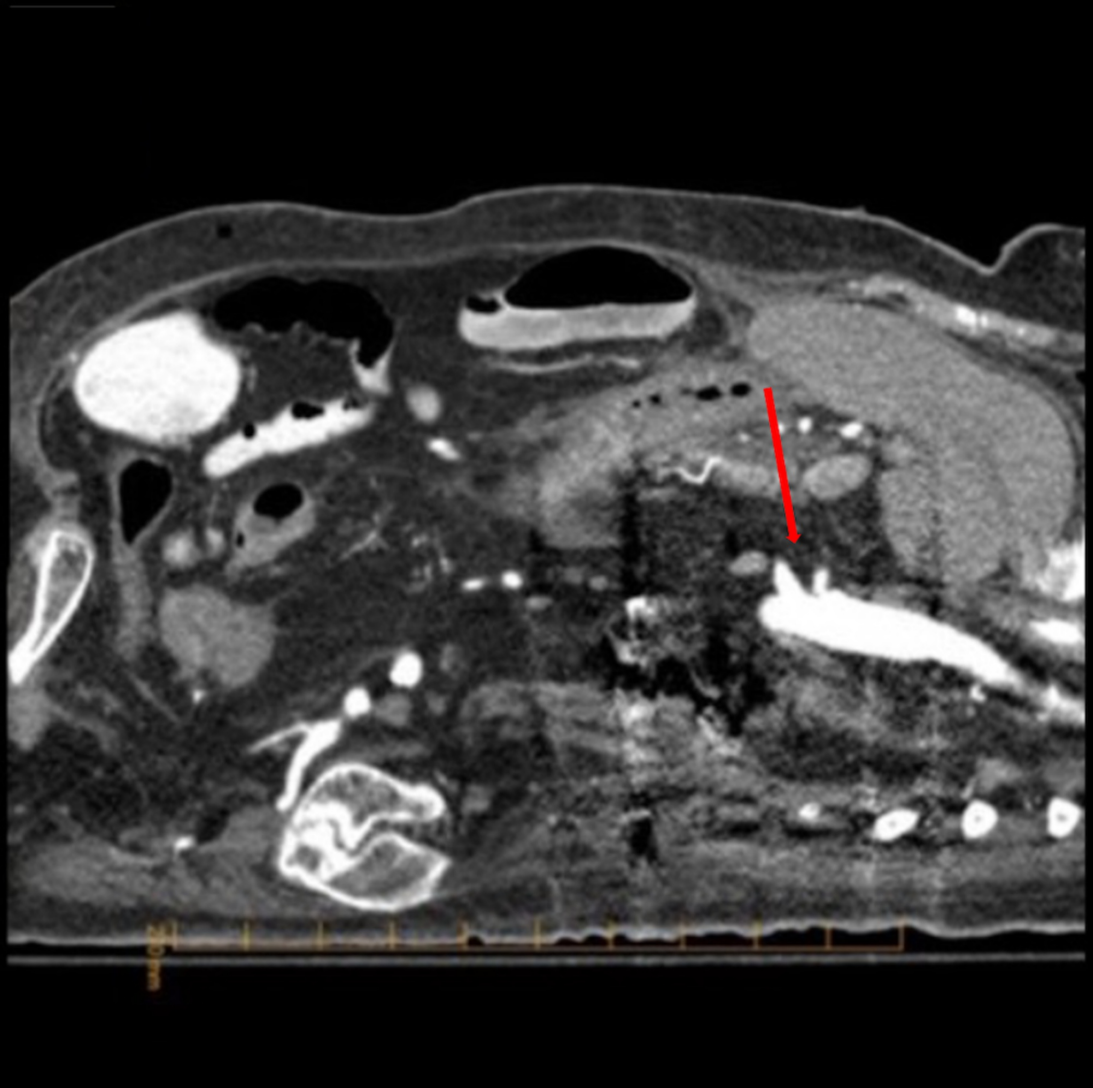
Computed tomography angiography showing a patent celiac and superior mesenteric artery
